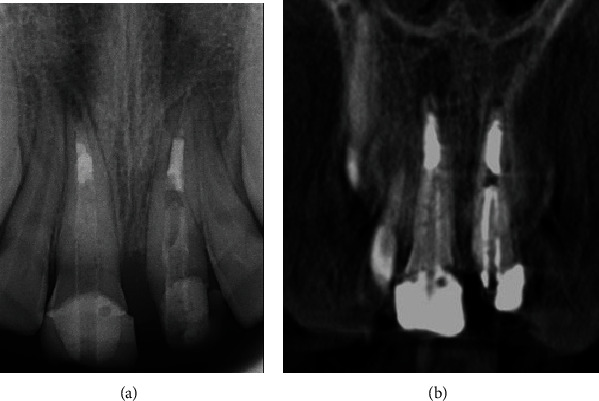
(a) Periapical and (b) CBCT radiographs showing periapical lesion related to tooth #11 and apical closure of tooth #21.
